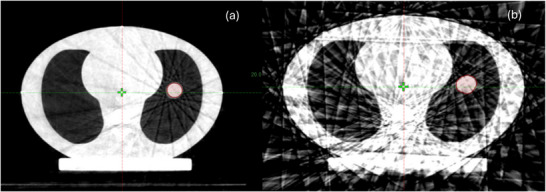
Display of 4DCBCT 0% phase image, reconstructed using (a) Advance algorithm with visibly less artifacts and (b) Basic algorithm, consisting of streak artifacts due to the smaller number of projections per phase [Window: −1000 to 0 HU].
